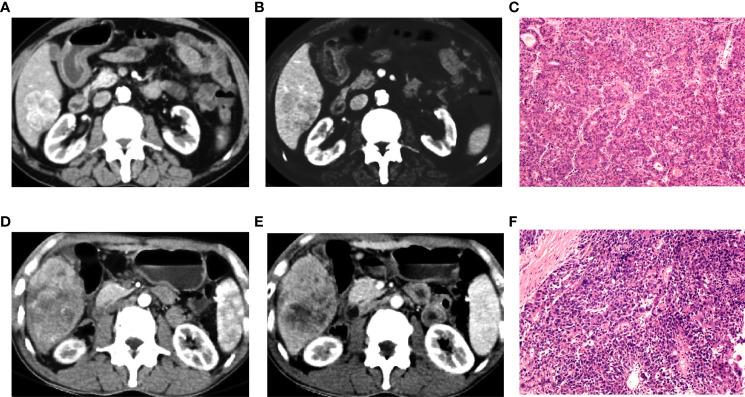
Preoperative contra-enhanced CT images and pathological pictures of two cases with hepatocellular carcinoma (HCC). (A–C) A 72-year-old man with non-early recurrence of HCC, the nomogram judged as low risk. (D–F) A 54-year-old man with early recurrence of HCC, the nomogram judged as high risk.
